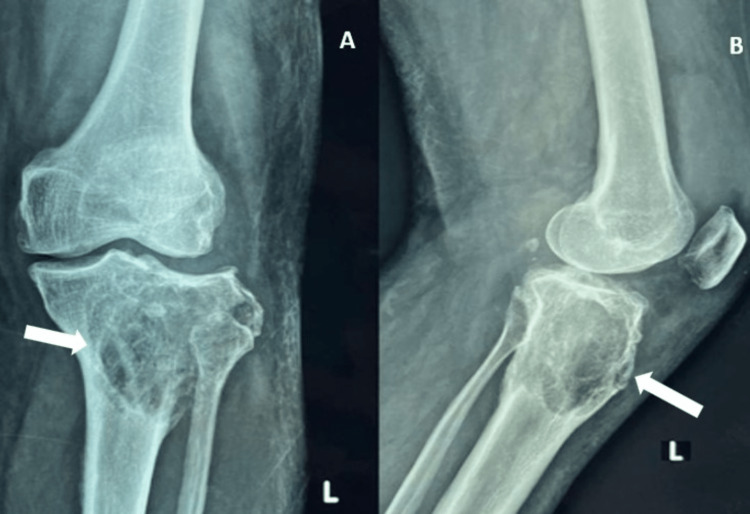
Anteroposterior (AP) and lateral view X-rays of the left knee showing osteolytic lesion involving the proximal tibia A and B are anteroposterior and lateral views of the left knee, showing bony swelling with a soap bubble appearance over the proximal third aspect of the tibia
